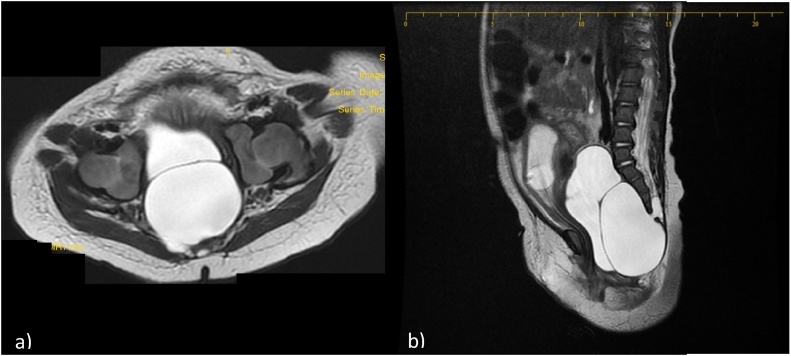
1a and 1b: Axial and sagittal T2-weighted images showing a septated cystic lesion located within the pelvis. This lesion is in continuity with the spinal canal through a hiatus located in the anterior and right aspect of the sacrum. (Horizontal line on the sagittal view). Dysraphism of the sacrum and coccyx is noted. Note that the urinary bladder contains a Foley catheter and is significantly compressed, as well as anteriorly and superiorly displaced.
